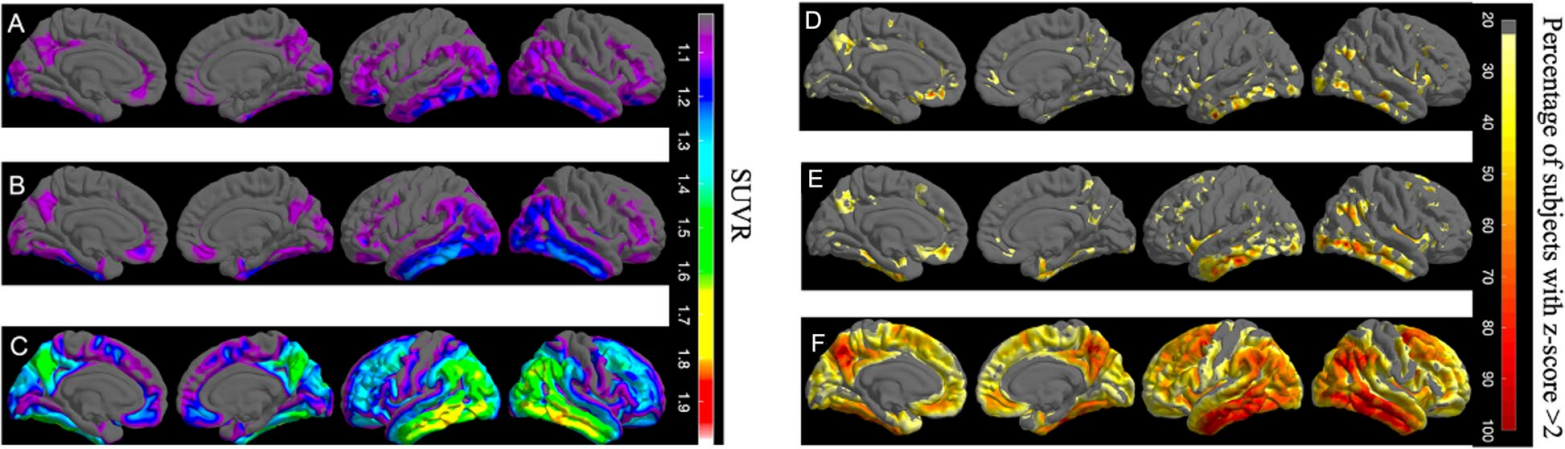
A The average SUVR surface map of B1 subjects, showing a lack of elevated flortaucipir signal in the entire cortex. B The average SUVR surface map of B2 subjects. There is an elevated flortaucipir signal primarily in the regions of *Eτ* VOI region with the exception of the left middle temporal gyrus. C The average SUVR surface map of B3 subjects. Widespread elevation in flortaucipir signal across the entire cortex with the exception of postcentral, precentral, and paracentral gyri and anterior cingulate. D Surface map showing the percentages of subjects that are tau-positive for all FreeSurfer regions in B1. E Surface map showing the percentages of subjects that are tau-positive for all FreeSurfer regions in B2. F Surface map showing the percentages of subjects that are tau-positive for all FreeSurfer regions in B3
